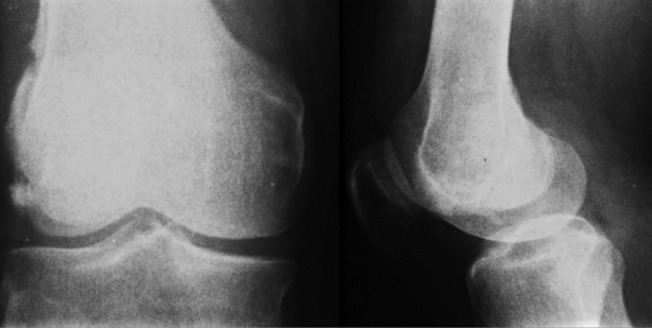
Pre-operative radiograph showing Hoffa fracture.
